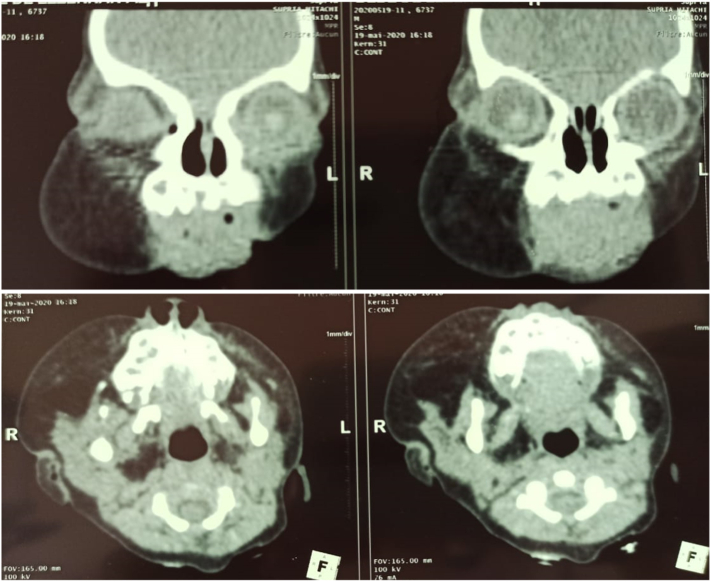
Axial and coronal sections of Facial CT scan showed a well-delimited homogeneous lesion measuring 54x50x28mm adjacent to the right mandibular cortex.
